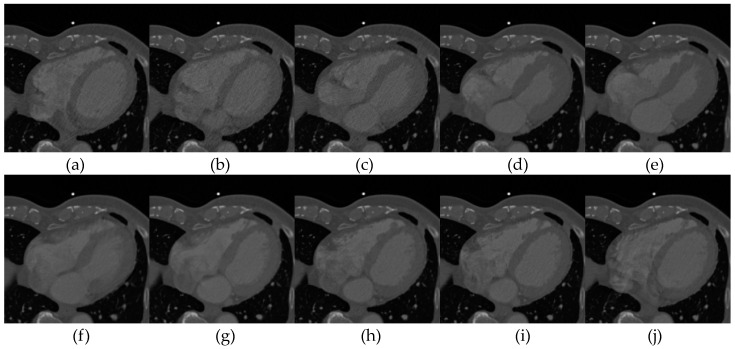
The axis slice of 10 3D cardiac CT images in one 4D sequence. (a–j) represent the 10 frames acquired from one whole cardiac cycle of one patient.
